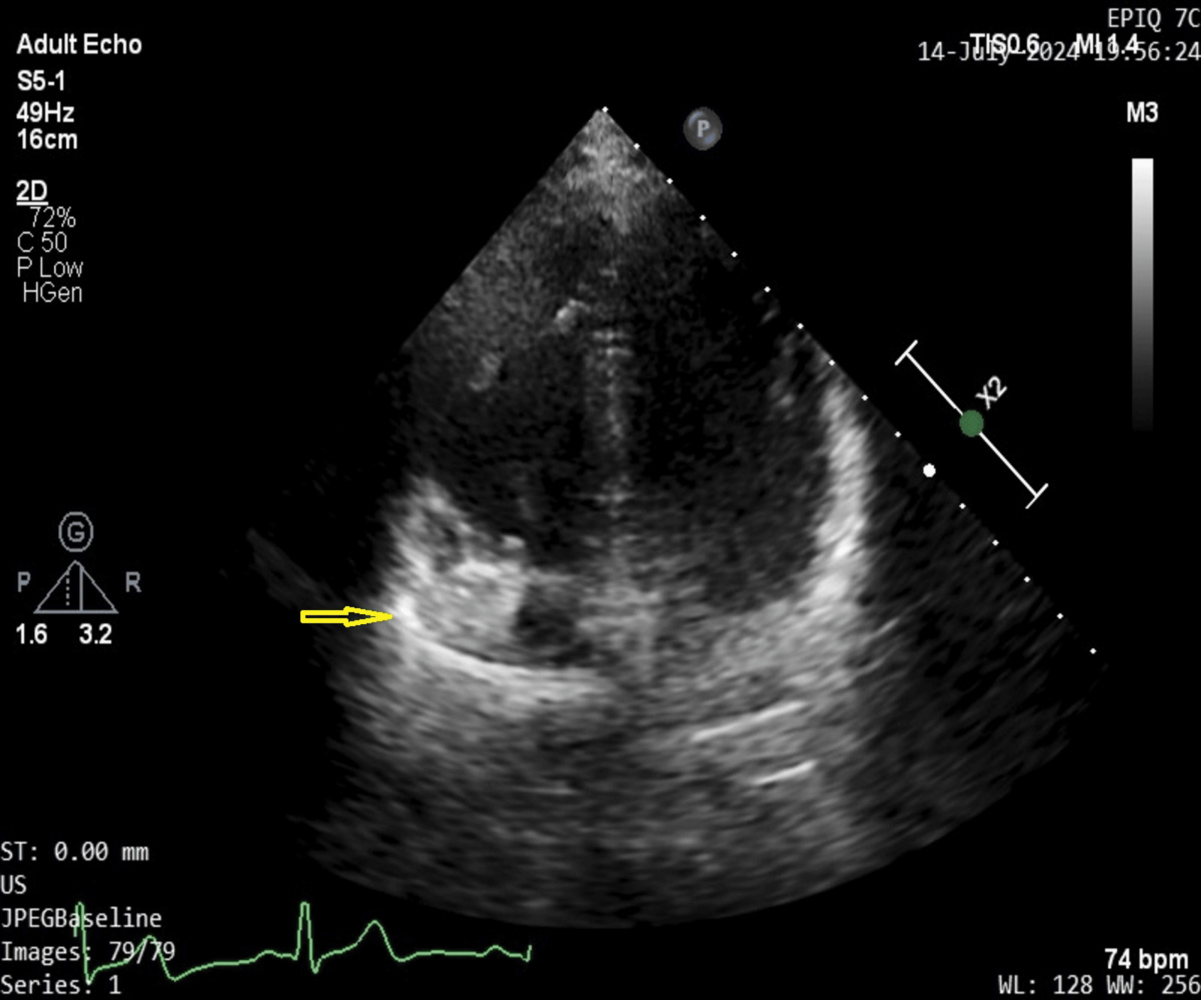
4-chamber view on transthoracic echocardiography showing hyperechoic mass (yellow arrow) in the right atrium.
